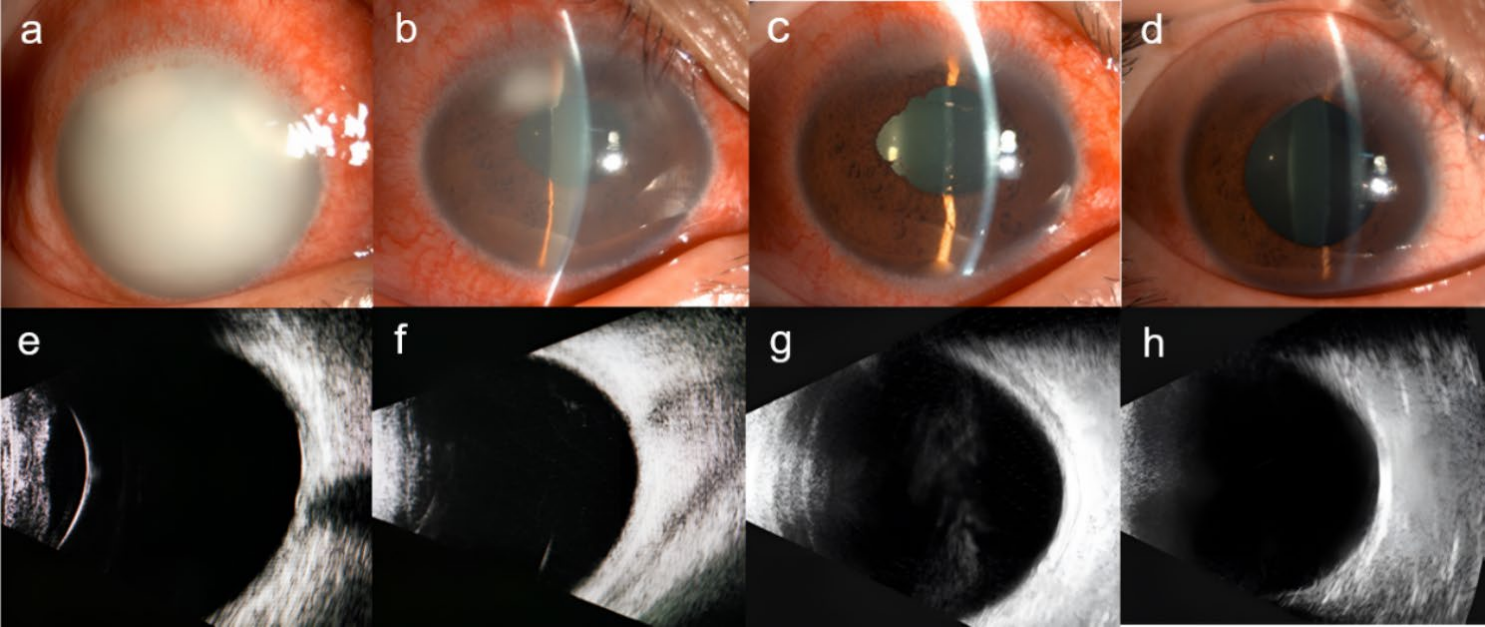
OD changes in uveitis. The top row shows eye segment photographs, and the bottom row shows eye ultrasound images on (a)(e). day 1, (b)(f). day 2, (c)(g). day 3, and (d)(h). day 10 of admission (discharge from hospital)
